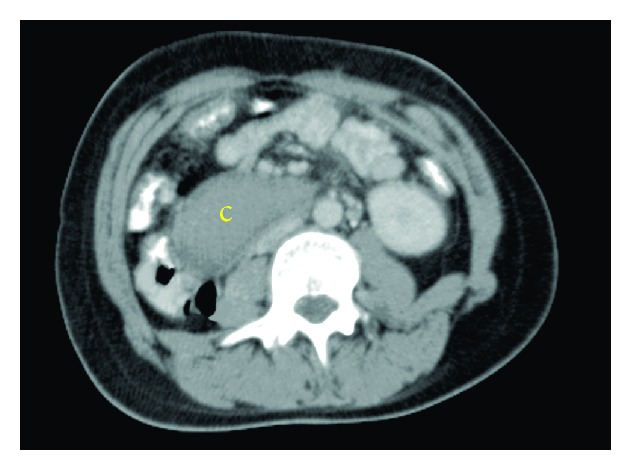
Oral and intravenous contrast-enhanced CT scan of the abdomen. The noncommunicating cyst extends along the third portion of the duodenum (c: cyst).
